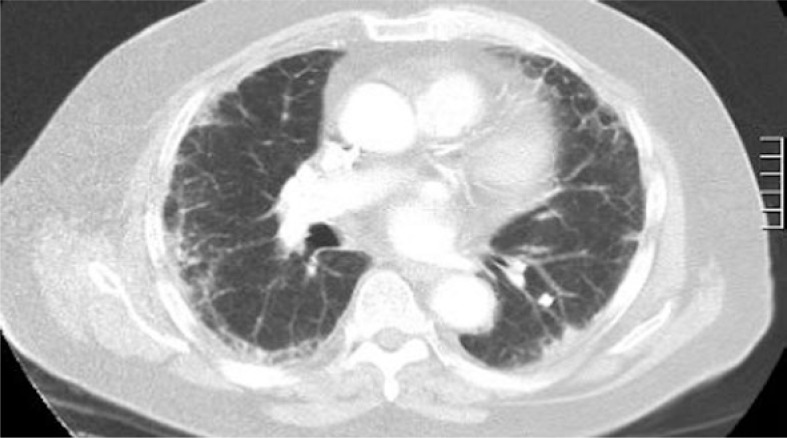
Representative image from re-staging CT January 2009; bilateral peripheral subpleural reticular changes and interlobular septal thickening without evidence of lung metastases.
